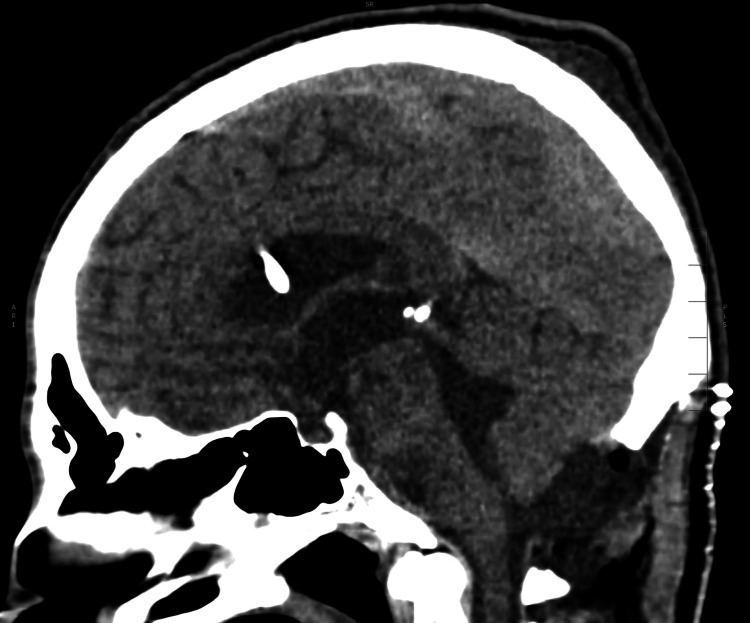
CT scan one week after surgery showing stark ventricle reduction. Fourth ventricle (#) now of normal size, with repositioning of the floor of the third ventricle (orange arrow). There was also reduction of the bowing of the medulla (white arrow). Usual post-surgical changes are also seen.
